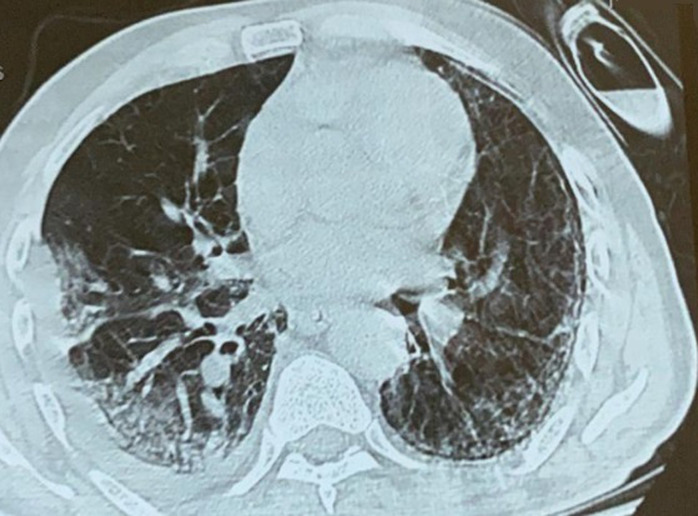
axial section of the computed tomography scan with lung parenchyma window showing a frosted glass appearance
